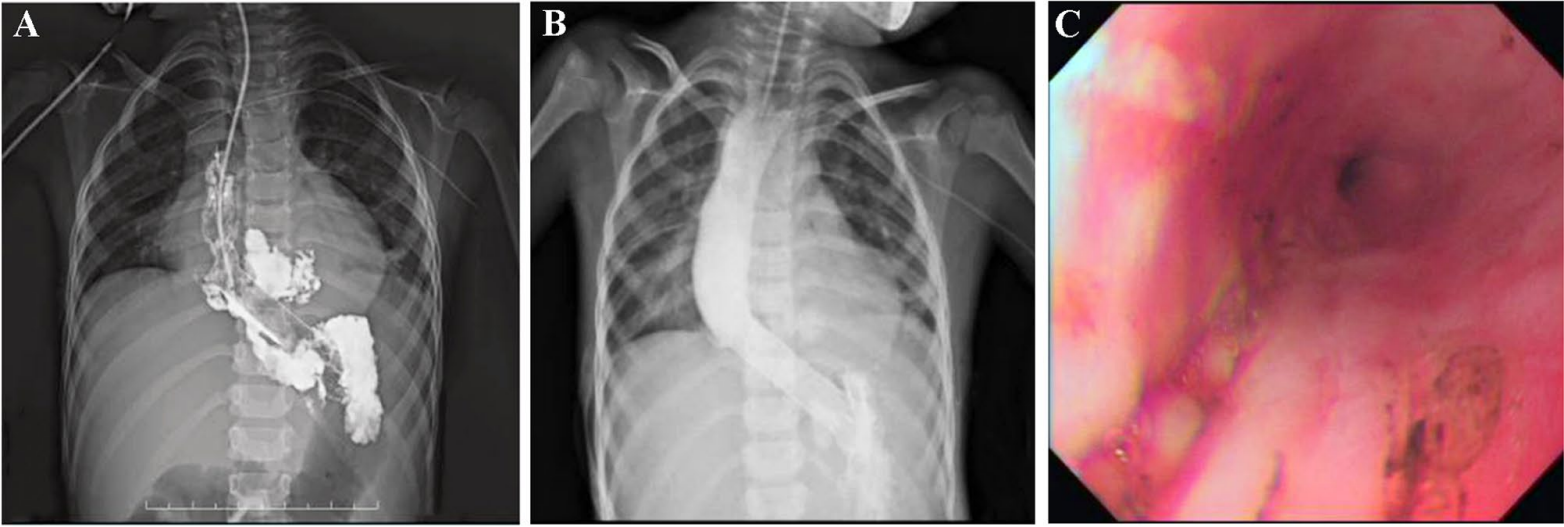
Stent placement in a patient with esophageal perforation after POEM (case 13). (A) A stent was placed after esophageal perforation was found during POEM, but stent migration occurred after 7 days; (B) After the stent position was adjusted under the endoscope, gastrointestinal tract radiography did not reveal extravasation of contrast medium; (C) The fistula was closed after the stent was removed 1.5 months later.
